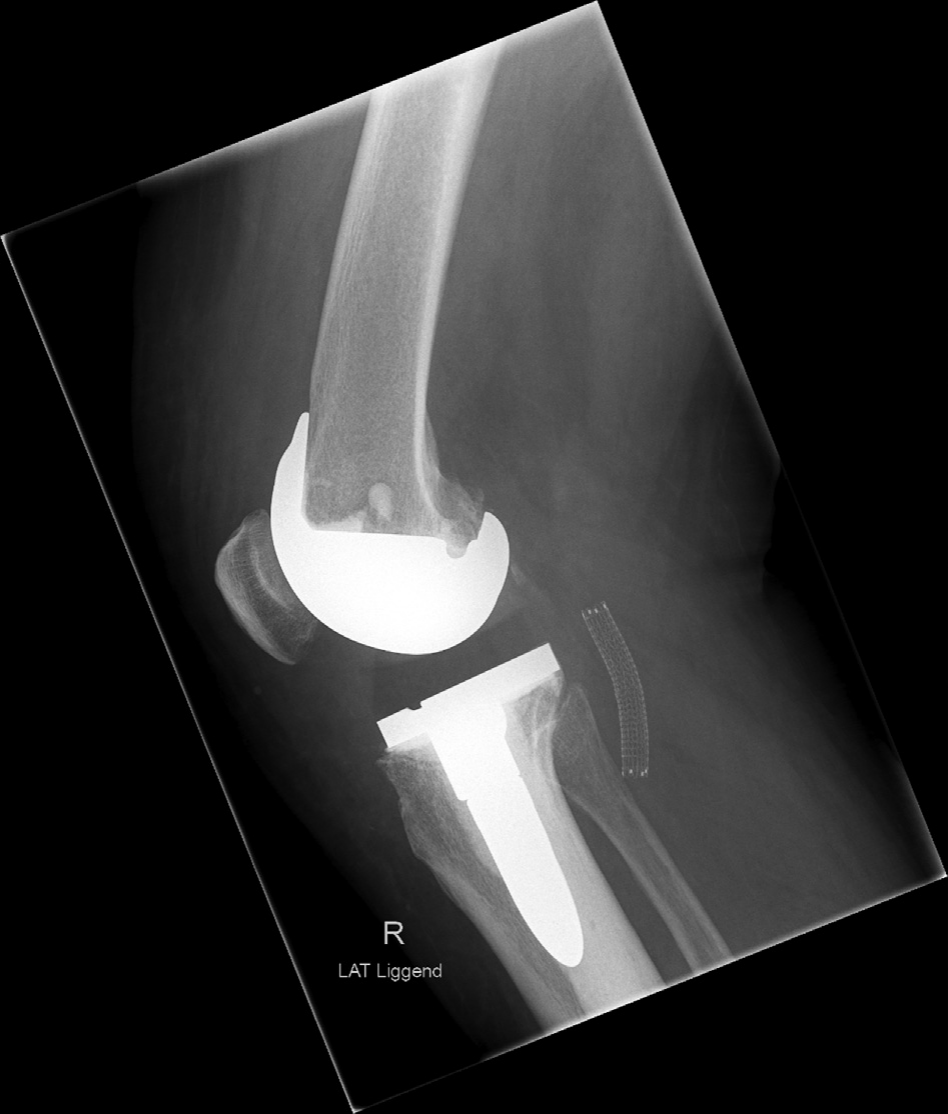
Postoperative sagittal radiograph after treatment showing the position of the stent graft.
